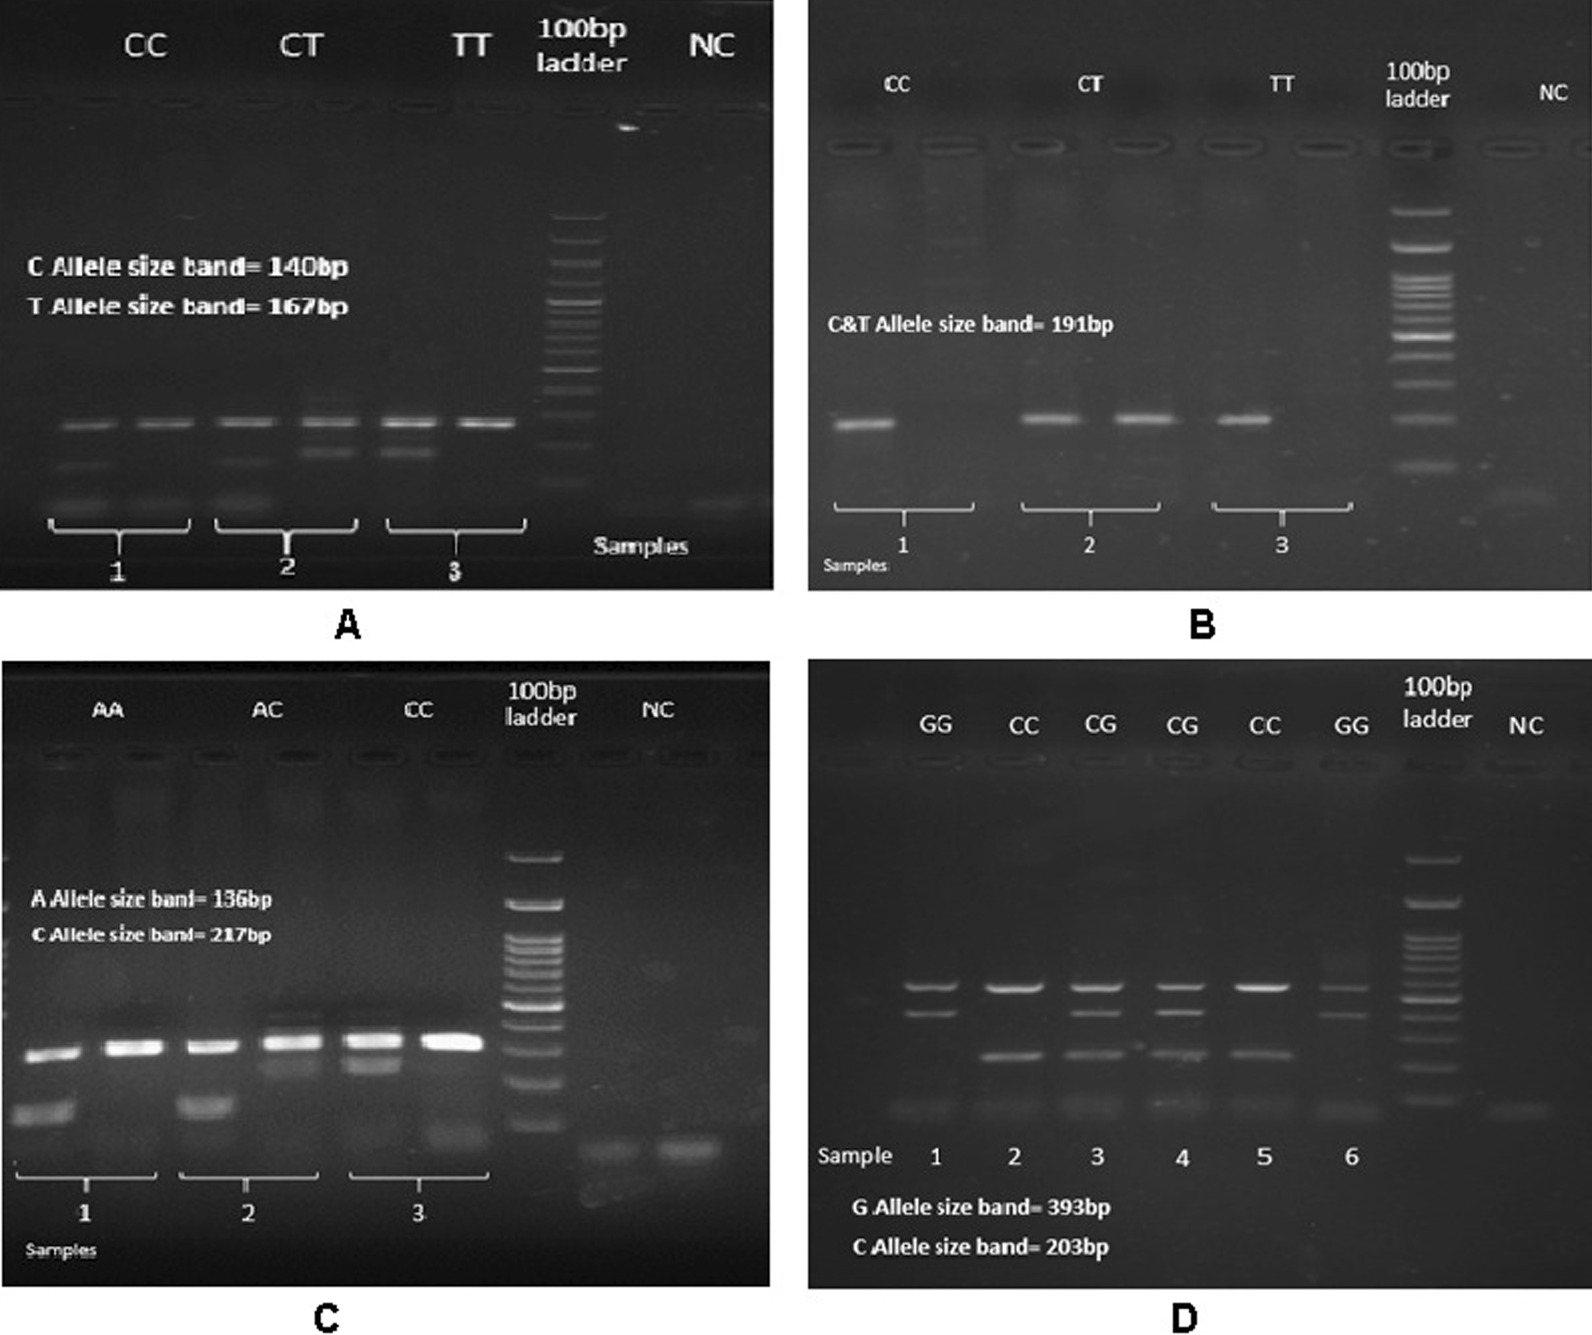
Agarose gel electrophoresis (2%) showing various genotypes of four polymorphisms as follows: A, Represent *rs4939827*, sample one; CC genotype (140 bp), sample 2; CT genotype (140, 167 bp) and Sample 3; TT genotype (167 bp). B, Represent* rs8085824*, sample one; CC genotype, sample 2; CT genotype and Sample 3; TT genotype(C & T Allel size band 191 bp). C, Represent *rs8088297*, sample one; AA genotype (136 bp), sample 2; AC genotype (136, 217 bp) and Sample 3; CC genotype (217 bp). D, Represent *rs34007497*, sample 1, 6; GG genotype (393 bp), sample 3, 4; CG genotype (203, 393 bp) and Sample 2, 5; CC genotype (203 bp)
